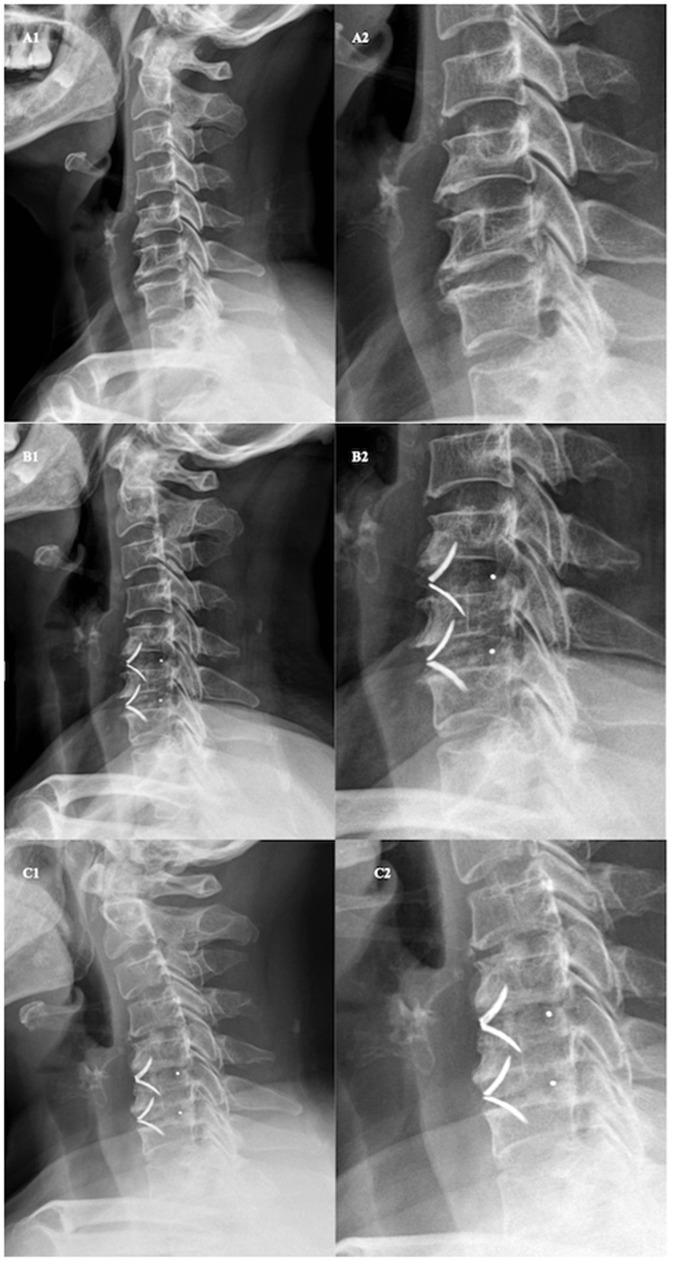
Demonstration of cage subsidence after ACDF and its influence on local and general cervical lordosis. Lateral radiograph (A1) and enhanced view (A2) before anterior cervical discectomy and fusion with ROI-C cage. Lateral radiograph (B1) and enhanced view (B2) one month after ACDF with ROI-C cage. Lateral radiograph (C1) and enhanced view (C2) one year after ACDF with ROI-C cage. Fused segment cobb angle were 0°, 10°, and, 0° and Cervical cobb angle were 2°, 5°, and −15° before operation, 1-month post-op, and 1-year post-op respectively.
